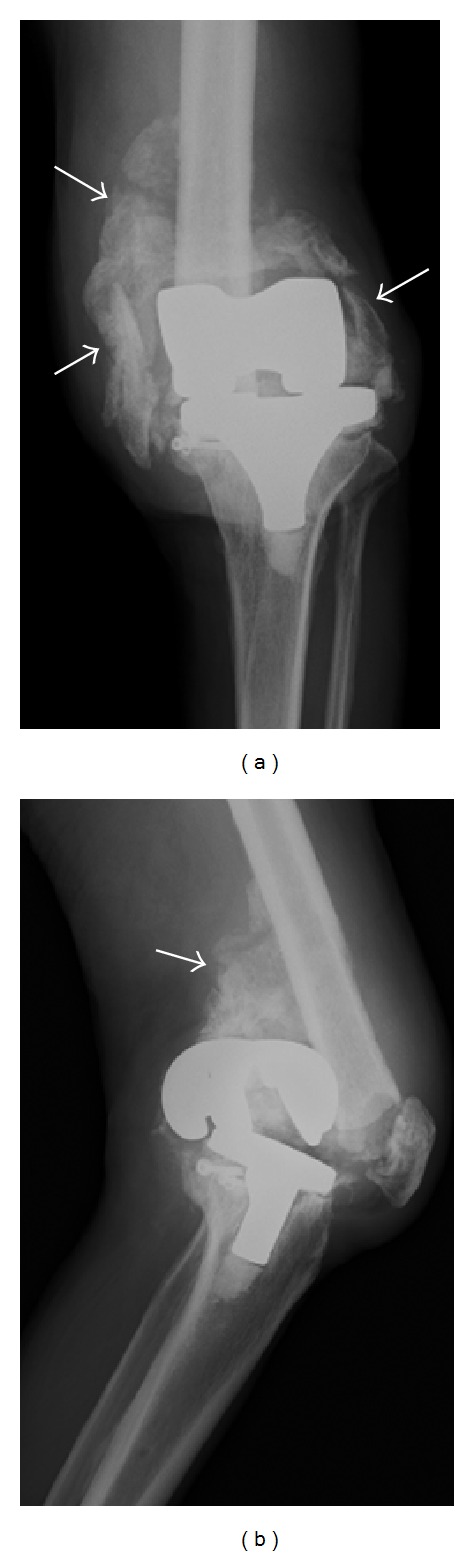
Radiographs of the left knee three months after the patient became unable to walk. Intra-articular bony fragments are enlarged and form giant ectopic ossified masses ((a), (b), arrows). Note that the femoral component is displaced and shifted posteriorly (b).
